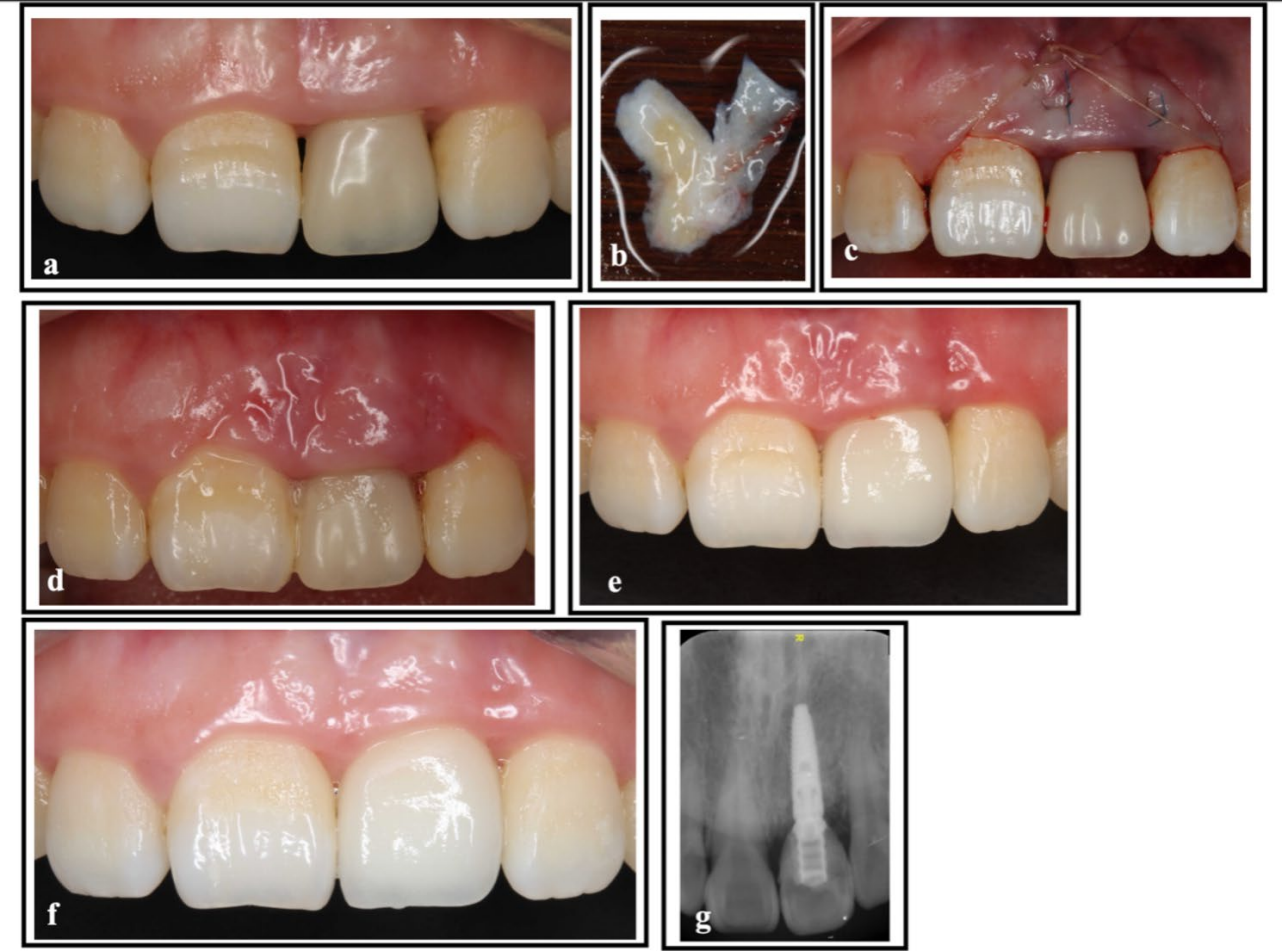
Surgical procedure and follow-up of Case 3. (a) Preoperative view showing papilla loss between central incisors and between the left maxillary central and lateral incisors. Note that the left maxillary central incisor is an implant. (b) Preoperative view of the CTG. (c) Postoperative view after suturing. (d) Suture removal 2 weeks after surgery. (e) A 3-month postoperative view of permanent ceramic crowns (f) At 12 months postsurgery, the papillae were completely recovered. (g) Radiographic image at baseline. CTG, connective tissue graft
